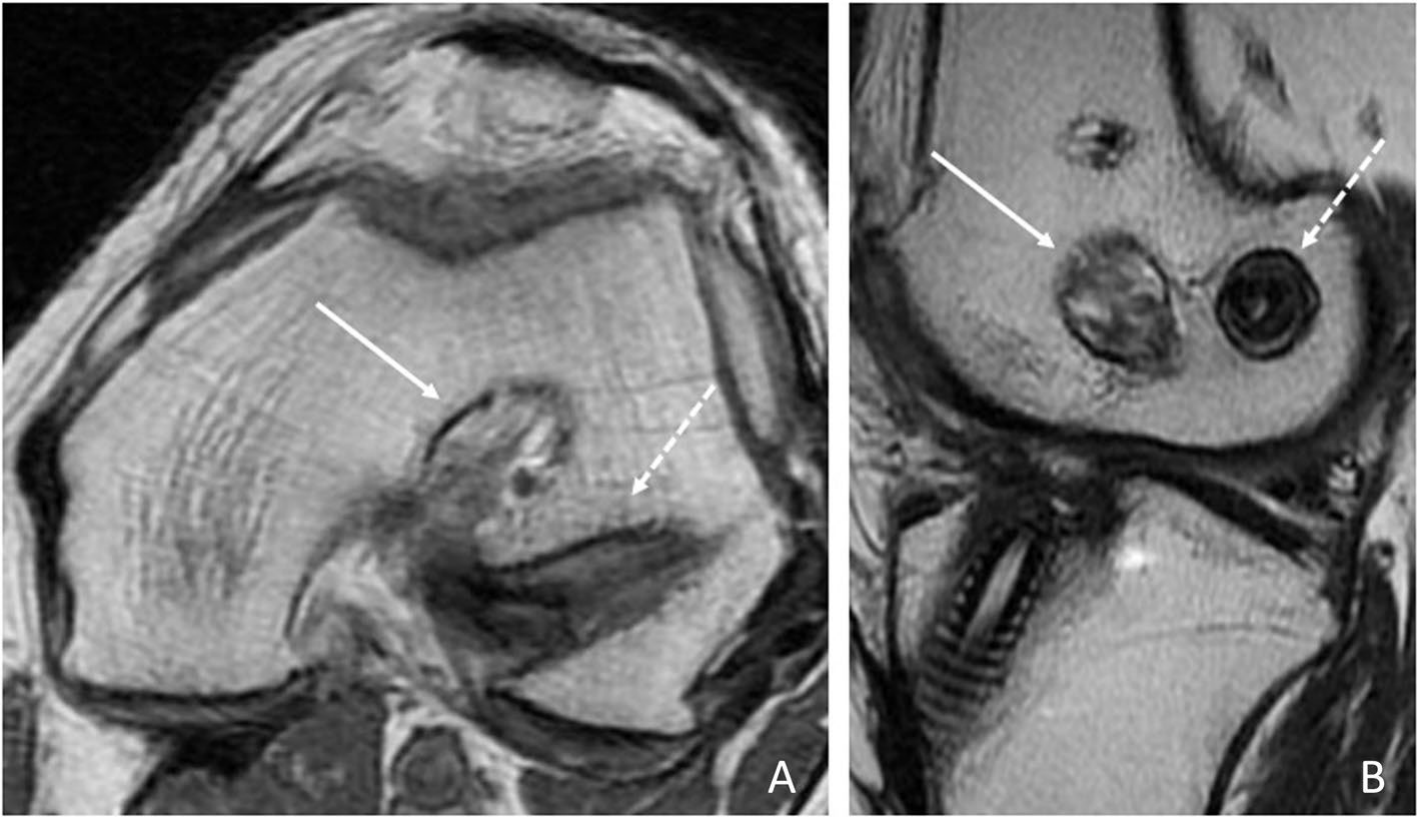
MRI axial (A) and sagittal (B) scans showing the case of a 24-year-old patient undergoing a previous non-anatomic ACL reconstruction with a transtibial technique (white arrow) and the new anatomic femoral tunnel placement (dotted line) using an anteromedial portal technique
